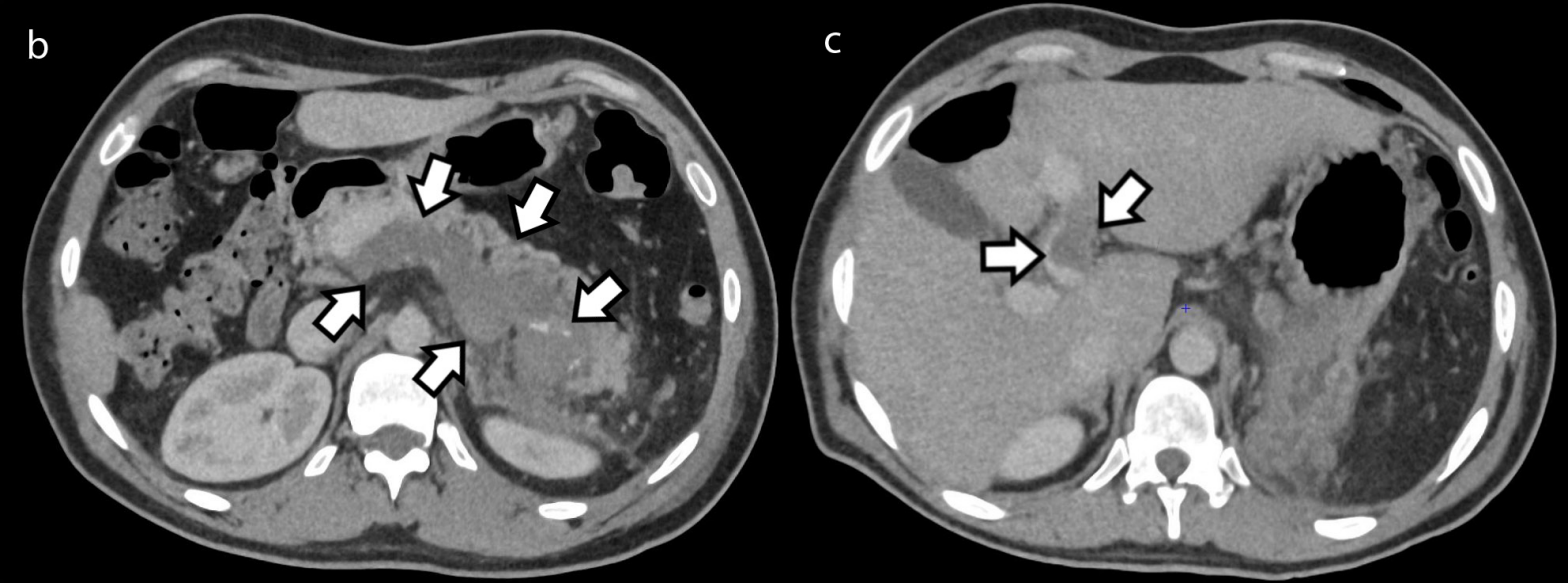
Axial contrast-enhanced portal phase CT slices at the level of the splenic vein (Figure 1b) and portal vein (Figure c) confirming a thrombosis of the splenic vein, extending into the portal vein as well as the part of the intrahepatic segment of the left branch of the portal vein (arrows).
